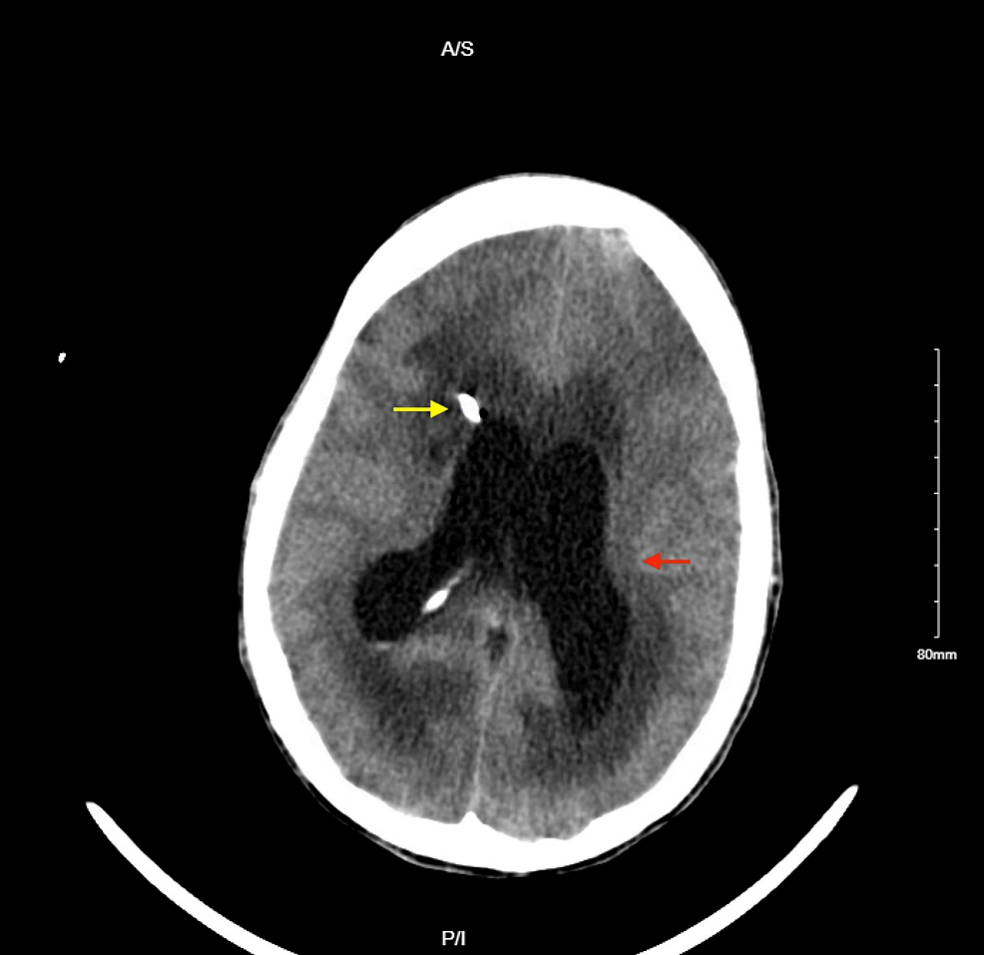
CT head obtained after the creation of ventriculoperitoneal shunt: stable positioning of the right transfrontal ventriculostomy catheter with stable marked vasogenic edema around the catheter tract (yellow arrow), as well as stable moderate dilation of the lateral ventricles (red arrow).
